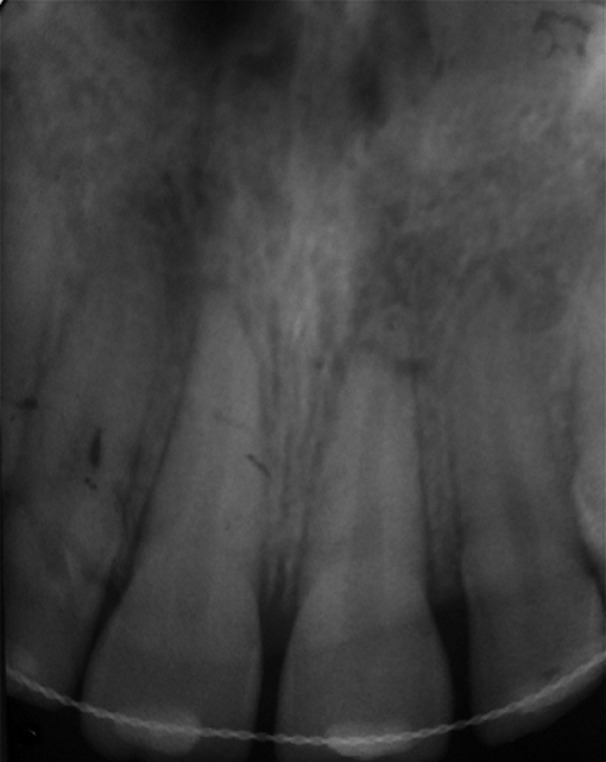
radiograph of splinted maxillary incisors immediately after injury
